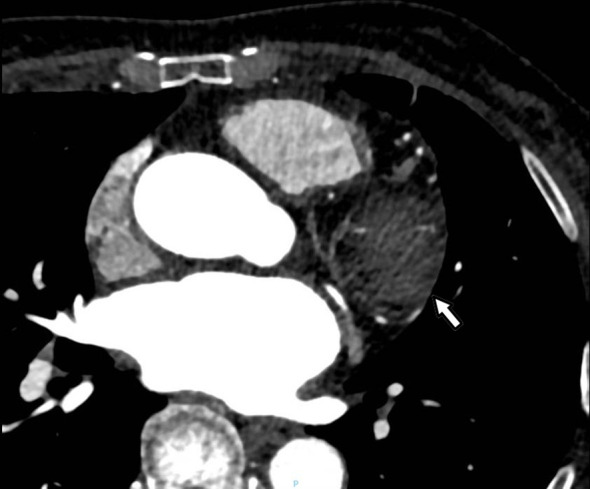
Coronary CTA confirmed a neoplastic pericardial tumor with a suspected coronary blood supply.
